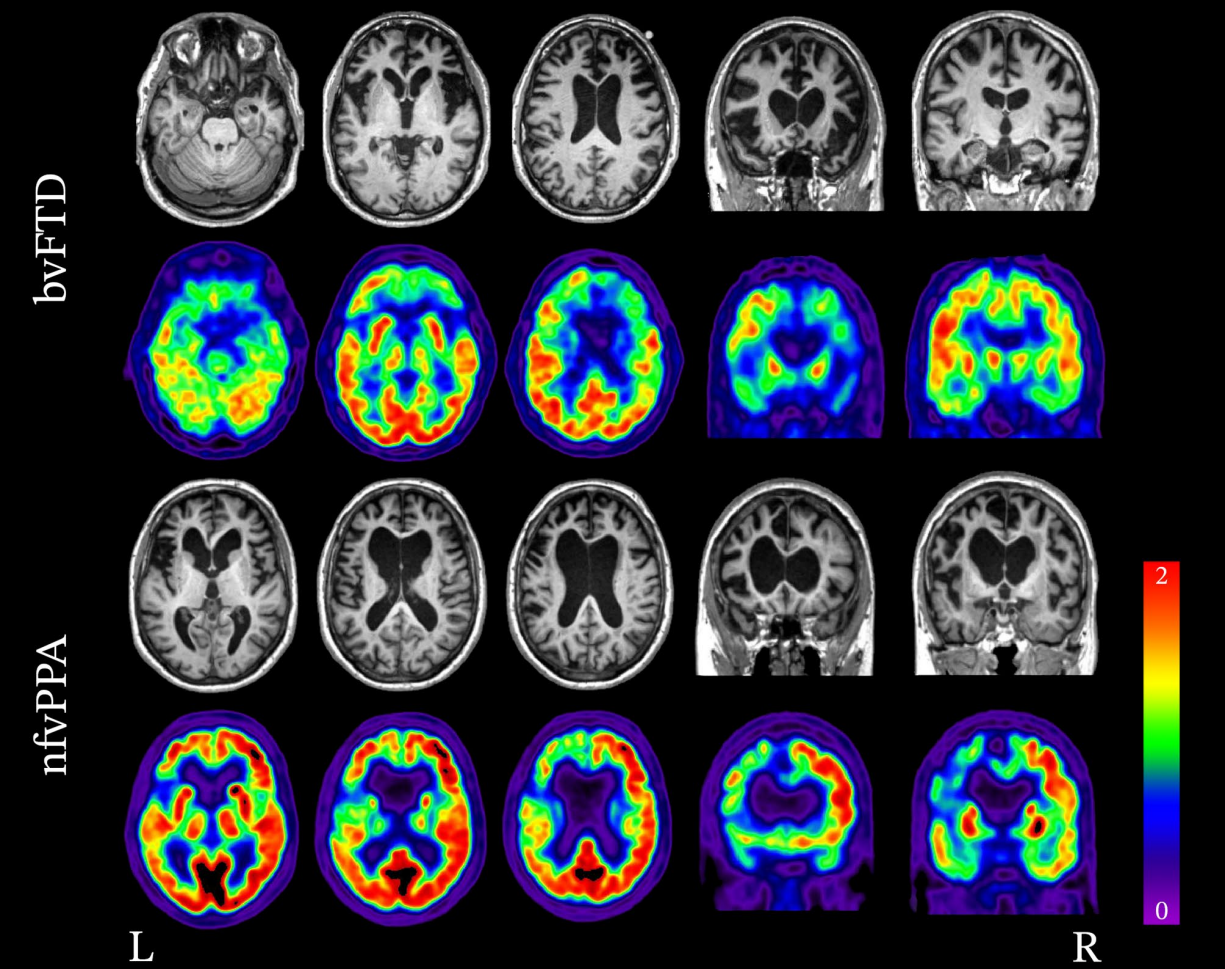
Neuroimaging patterns associated with Pick’s disease (FTLD-tau, 3R). Structural MRI and FDG-PET demonstrating the variability in patterns of atrophy and hypometabolism attributed to Pick’s disease (3R tau) neuropathology. In the case of bvFTD, significant bilateral frontal lobe atrophy and hypometabolism is seen. In the case of nfvPPA, atrophy and hypometabolism is lateralized and is greatly impacting the left frontal lobe more so than the right.
